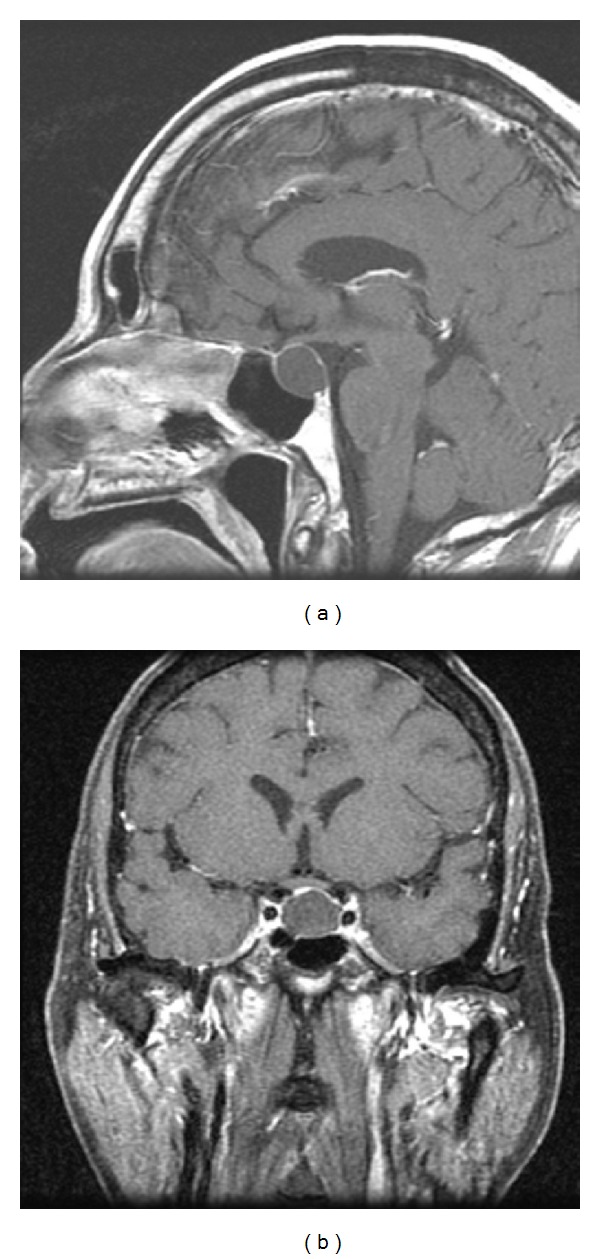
Sagittal and coronal postcontract MRI in late June revealing a sellar-suprasellar mass with some optic compression.
